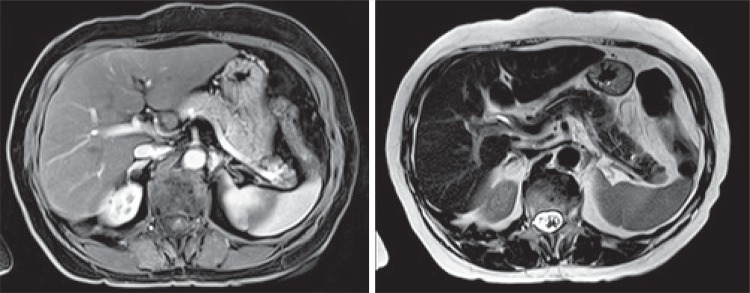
Abdominal magnetic resonance imaging (T1 and T2)
